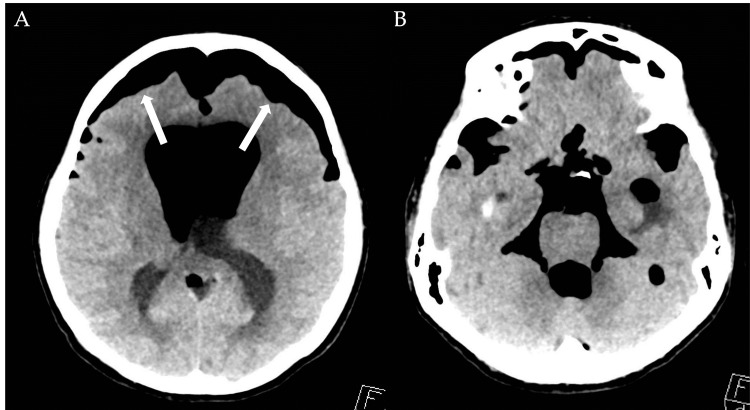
Non-contrast CT brain in the axial cuts, showing extensive pneumocephalus with air in the subarachnoid space and intraventricular space along with “twin-peak” sign (white box arrows), suggestive of tension pneumocephalus.
